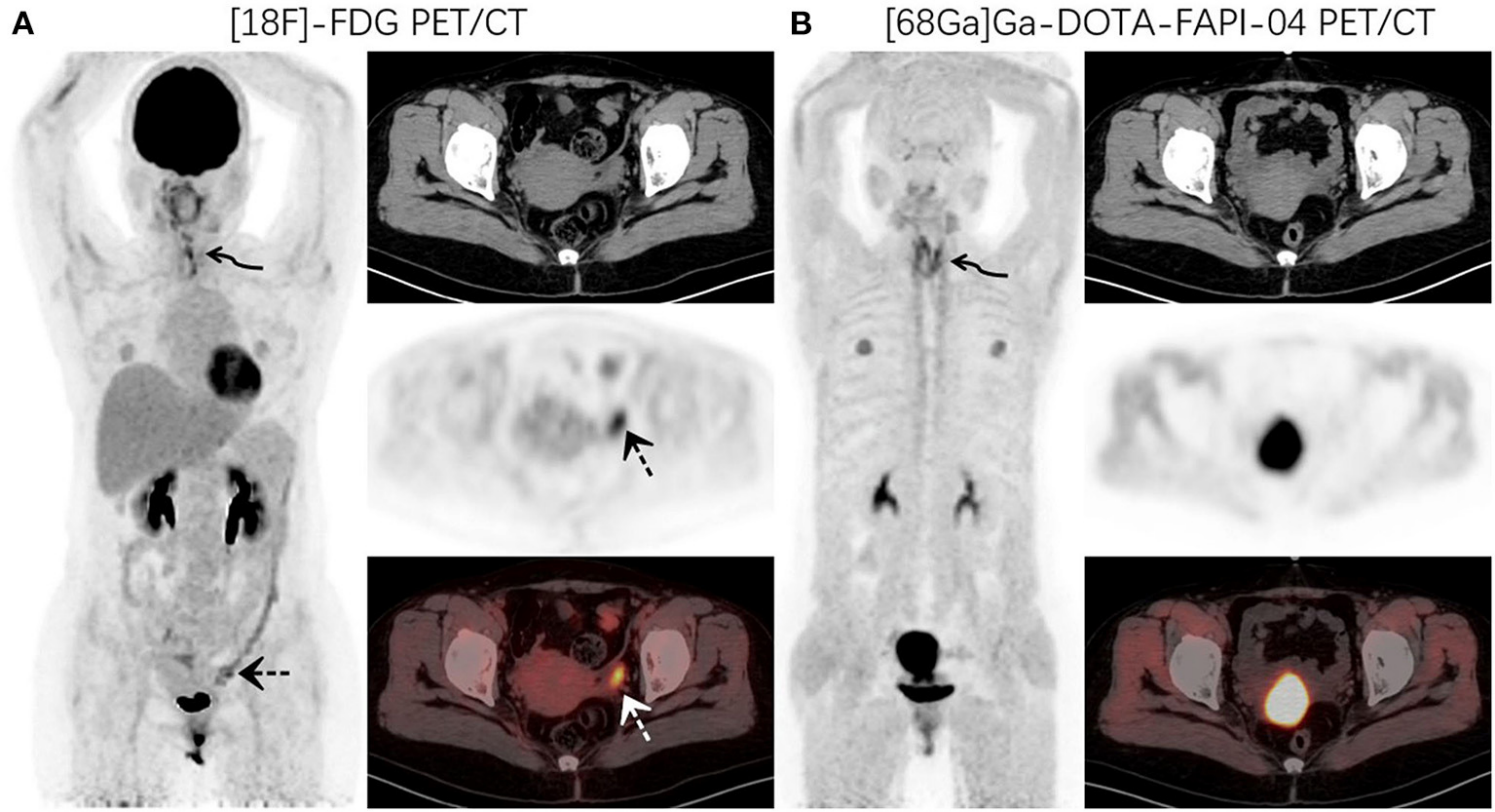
PET/CT was performed for restaging in a 52-year-old woman who underwent thyroidectomy for thyroid cancer 1 year prior. ^18^F-FDG PET/CT (A) images showed increased FDG uptake in the thyroidectomy area (curved arrow, SUVmax7.4) and left adnexal region (dotted arrow, SUVmax7.0). ^68^Ga-DOTA-FAPI-04 PET/CT (B) images demonstrated increased uptake of imaging agents in the thyroid area (curved arrow, SUVmax8.9), but no abnormal uptake was observed in the left adnexal region.
